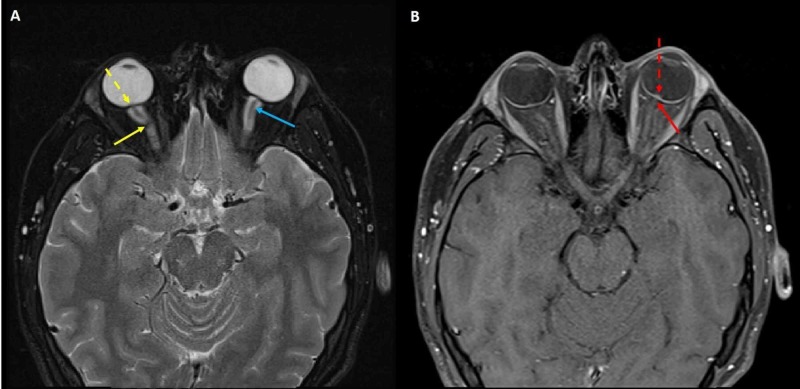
Magnetic resonance imaging (MRI) of the orbits with gadolinium contrast demonstrating papilledema (A) Axial T2W showing flattening of the posterior sclera (dashed yellow arrow), distension of the peri-optic subarachnoid space (solid blue arrow) and vertical tortuosity of the orbital optic nerve (solid yellow arrow) and (B) Axial T1W with contrast showing enhancement of the prelaminar optic nerve (solid red arrow) and intraocular protrusion of the prelaminar optic nerve (dashed red arrow).
